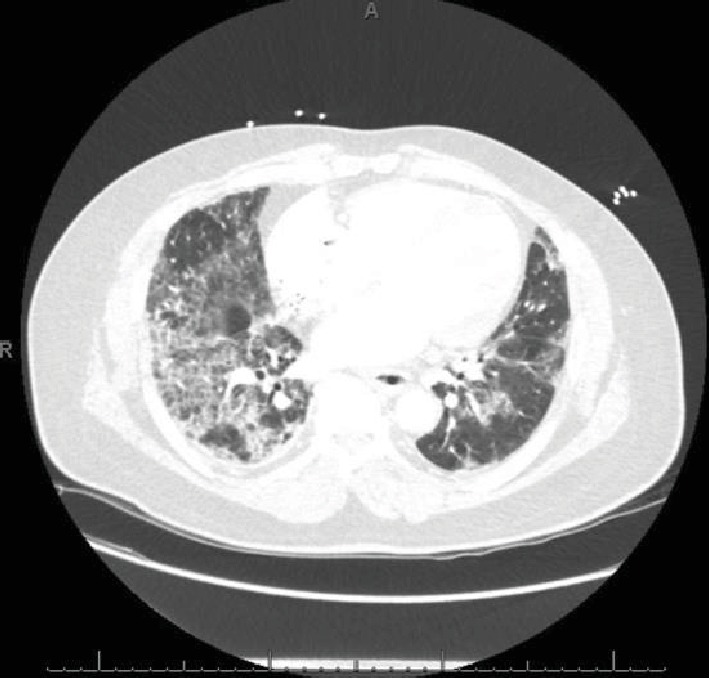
CT chest w/ contrast, lung window, showing initial acute pneumonitis findings: extensive ground-glass opacities and interlobular septal thickening with bilateral traction bronchiectasis. Pulmonary nodules are not well seen due to ground-glass opacities.
